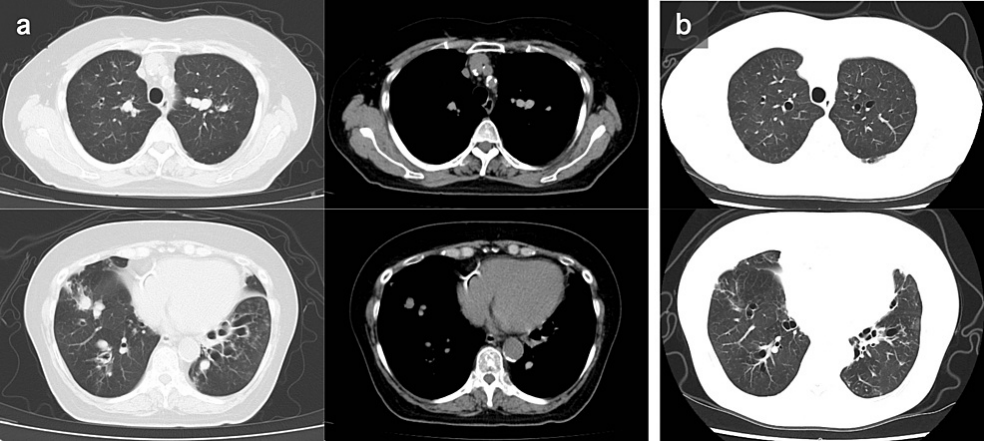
Chest computed tomography (CT) findings. (A) Chest CT findings at the first visit. High-attenuation mucus (HAM) plugs in the right upper lobe and bilateral lower lobes. (B) Chest CT findings after 12 weeks of treatment with dupilumab. The HAM plugs in both lungs have disappeared.
